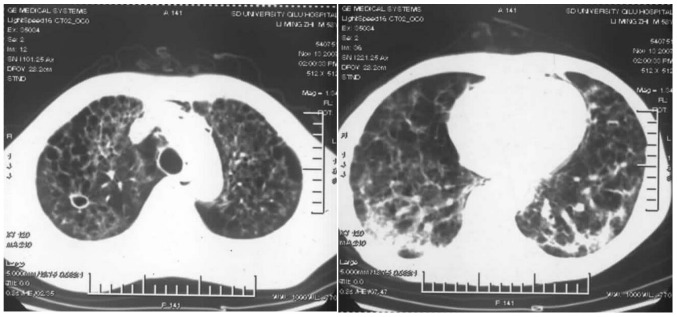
Chest computed tomography on November 10, 2007: The diffuse reticular, patchy, nodular shadows (evident in the central region) have been reduced and the cystic wall has thinned.
